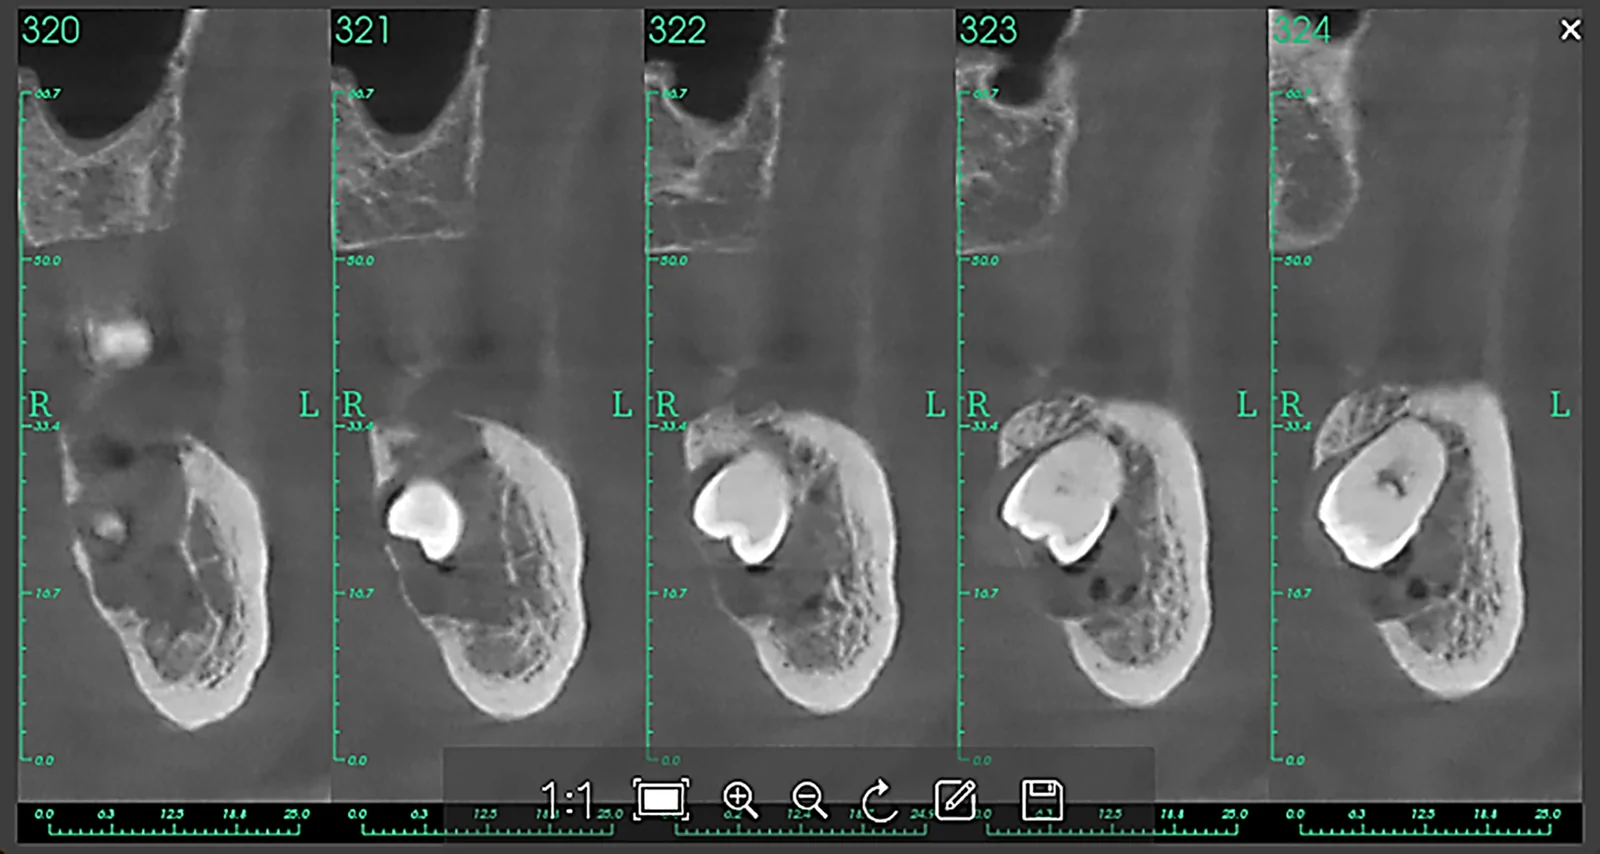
CBCT scan showing perforation of the lingual cortical plate and invasion of the left mandibular neurovascular bundle by the lesion. CBCT = cone-beam computed tomography.
